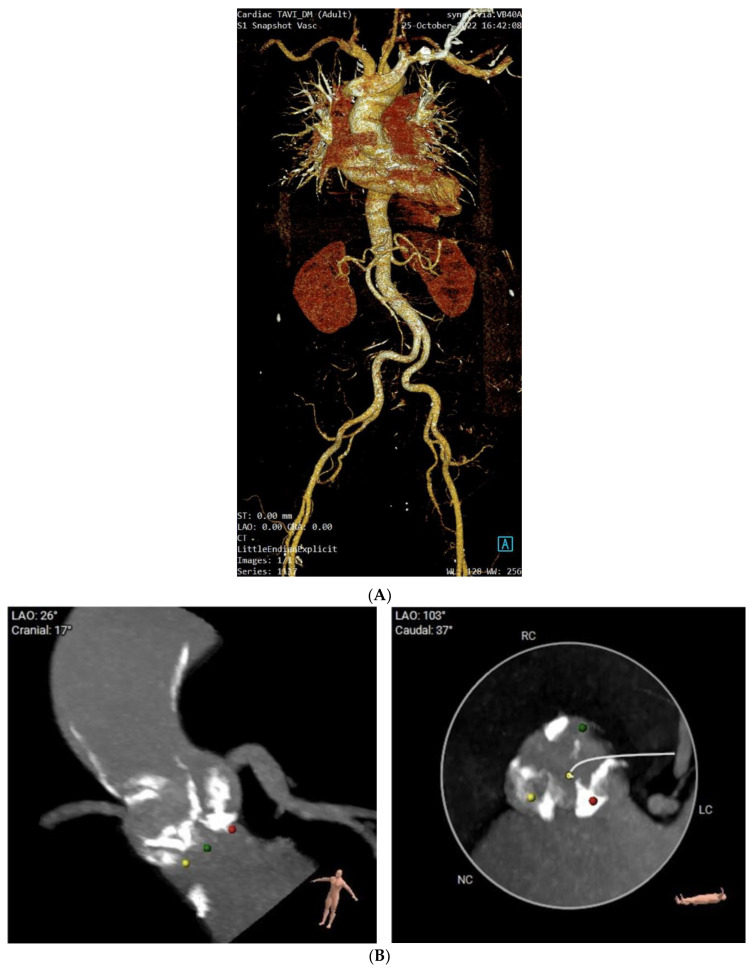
(A). Computed tomography angiography. (B). Moderate aortic valve calcifications.
